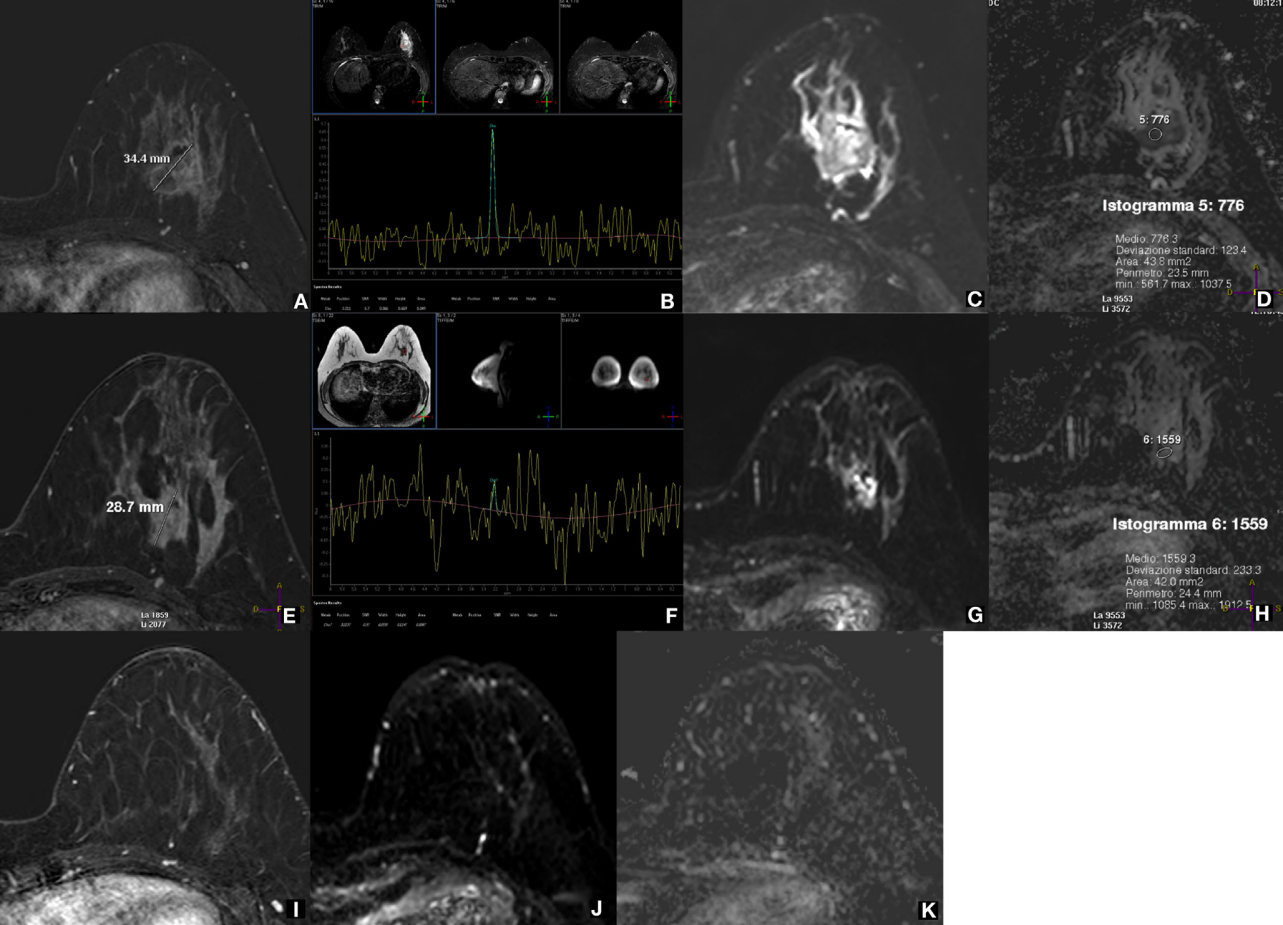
1H-MRS and DWI for evaluating the response to neoadjuvant therapy (NAT) of a locally advanced breast cancer. A 37-year-old woman with locally advanced breast cancer before, during, and after neoadjuvant therapy (NAT). Before treatment, the lesion at the lower external quadrant of the left breast is well depicted as a 34.4-mm nodule on subtracted CE-MRI with 0.1 mmol/kg of gadobenate dimeglumine (A), shows a high tCho peak at 1H-MRS with a signal-to-noise ratio (SNR) of 6.7 (B), low diffusivity as a high signal on DWI (C), and a low (0.776 × 10^−3^ mm^2^/s) mean ADC value (D). After two NAT cycles, the lesion is reduced in size (28.7 mm) at CE-MRI (E), while the tCho peak is no longer detectable at 1H-MRS (F); DWI (G) and ADC map (H) show an evident increased diffusivity (mean ADC 1.559 × 10^−3^ mm^2^/s). After the end of treatment, the lesion is not visible at all on CE-MRI (I), DWI (J), and ADC map (K); therefore, MRS was not performed. The lesion was a metaplastic carcinoma with condroid differentiation (negative for estrogen, progesterone, and HER2 receptors, Ki67 80%), and after surgical removal, a complete pathological response was appreciated. Both 1H-MRS and DWI early predicted the pathological response to NAT showing an effect more pronounced than that of CE-MRI. Acquisition times: CE-MRI 9 min; 1H-MRS 8 min (including preparation); DWI 5 min (Philips Achieva STx 3.0 T, MultiTransmit radiofrequency technology; dedicated 16-channel breast coil; Azienda Ospedaliera Universitaria Integrata, Verona, Italy).
